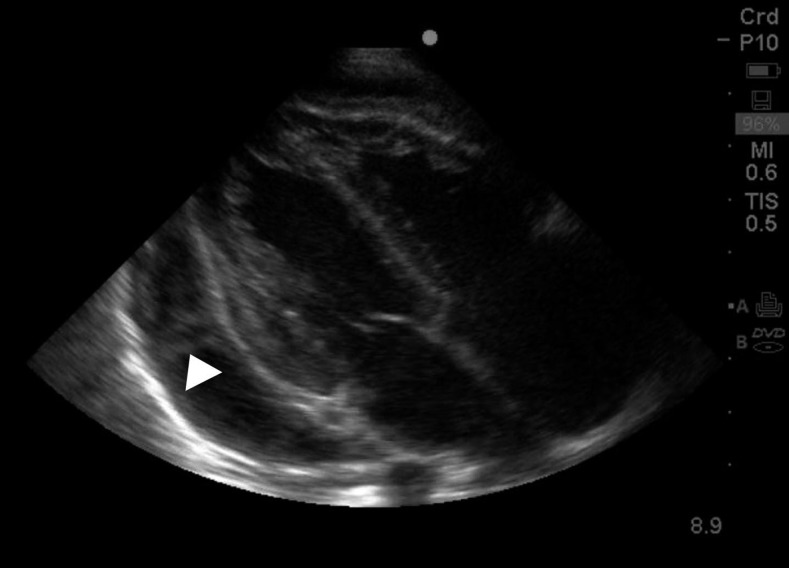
Parasternal long axis view showing moderate pericardial effusion and fibrinous exudate (arrow).
